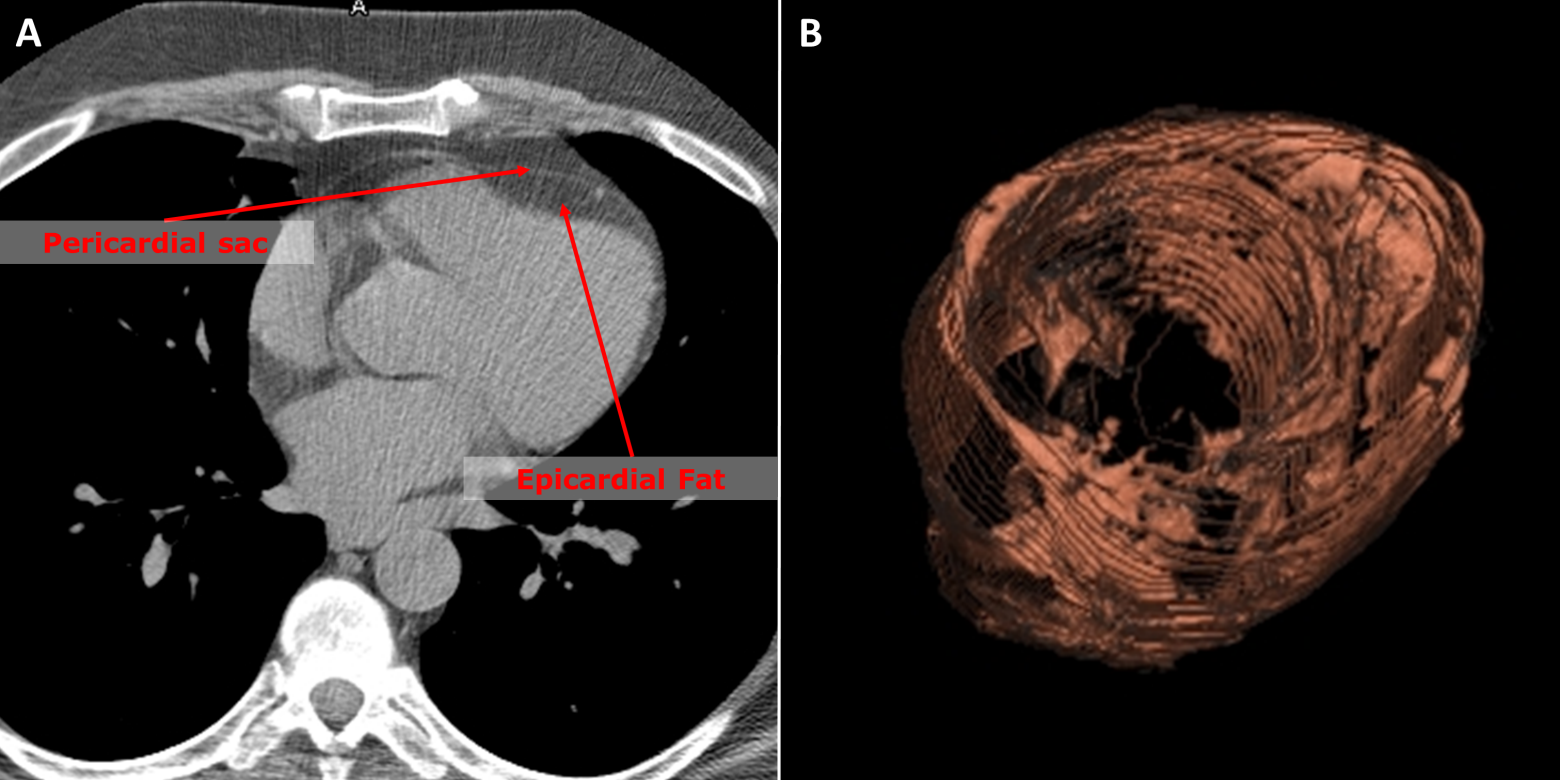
Epicardial fat quantification from cardiac CT examination. The pericardial sac was manually traced in axial images as region of interest (A). Within this region of interest, pixels between -195 and -45 Hounsfield Units were accounted as fat. After 3-dimensional reconstruction, EAT volume was calculated by summation of all pixels accounted as fat (B). EAT attenuation was defined as mean Hounsfield Units of all fat pixels of the EAT volume.
